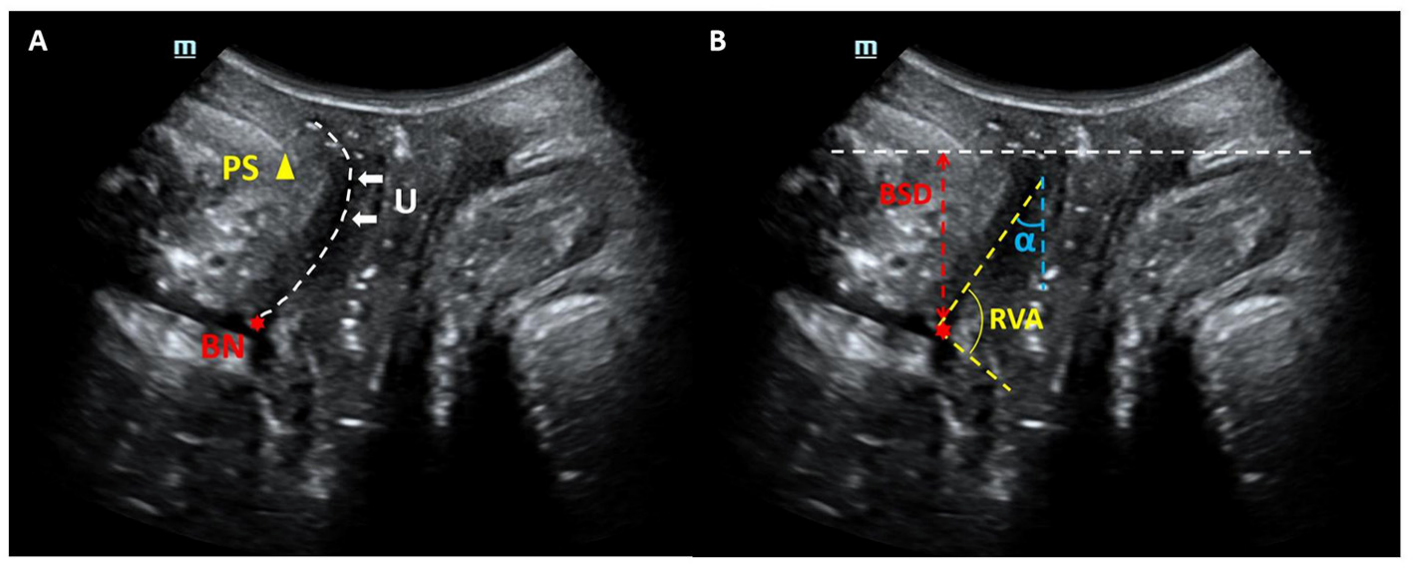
Mid-sagittal ultrasound images of the pelvic organs. (A) Anatomical landmarks: the public symphysis (PS), bladder neck (BN), and urethra (U) are also visualized. (B) Index measurement mark: bladder symphyseal distance (BSD), urethral axis angle (α angle) and retrovesical angle (RVA).
